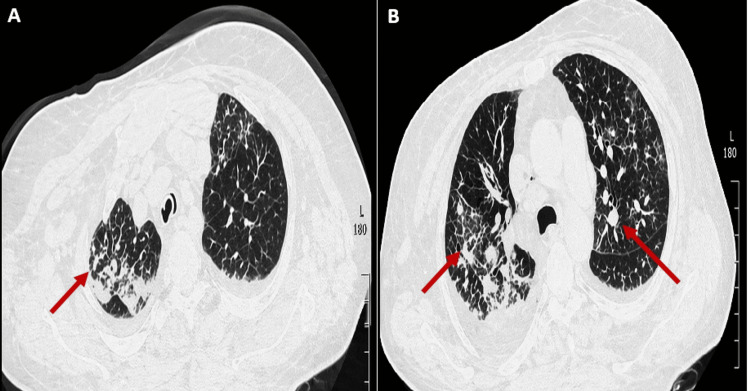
Axial thoracic CT scans (A and B) showing bronchiectasis, indicated by arrows
